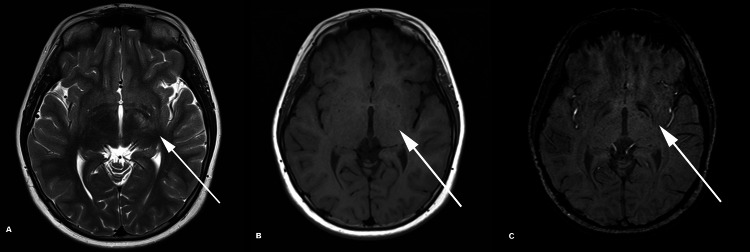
MRI in axial planes showing T2-weighted (A), T1-weighted (B), and SWI images (C). An ill-defined patchy hyperintense signal on T2-weighted (A, white arrow) and an isointense signal for cortical gray matter on T1-weighted images can be seen (B, white arrow). On SWI (C), an associated blooming artifact of the same region can be seen (white arrow). MRI: magnetic resonance imaging; SWI: susceptibility-weighted imaging
